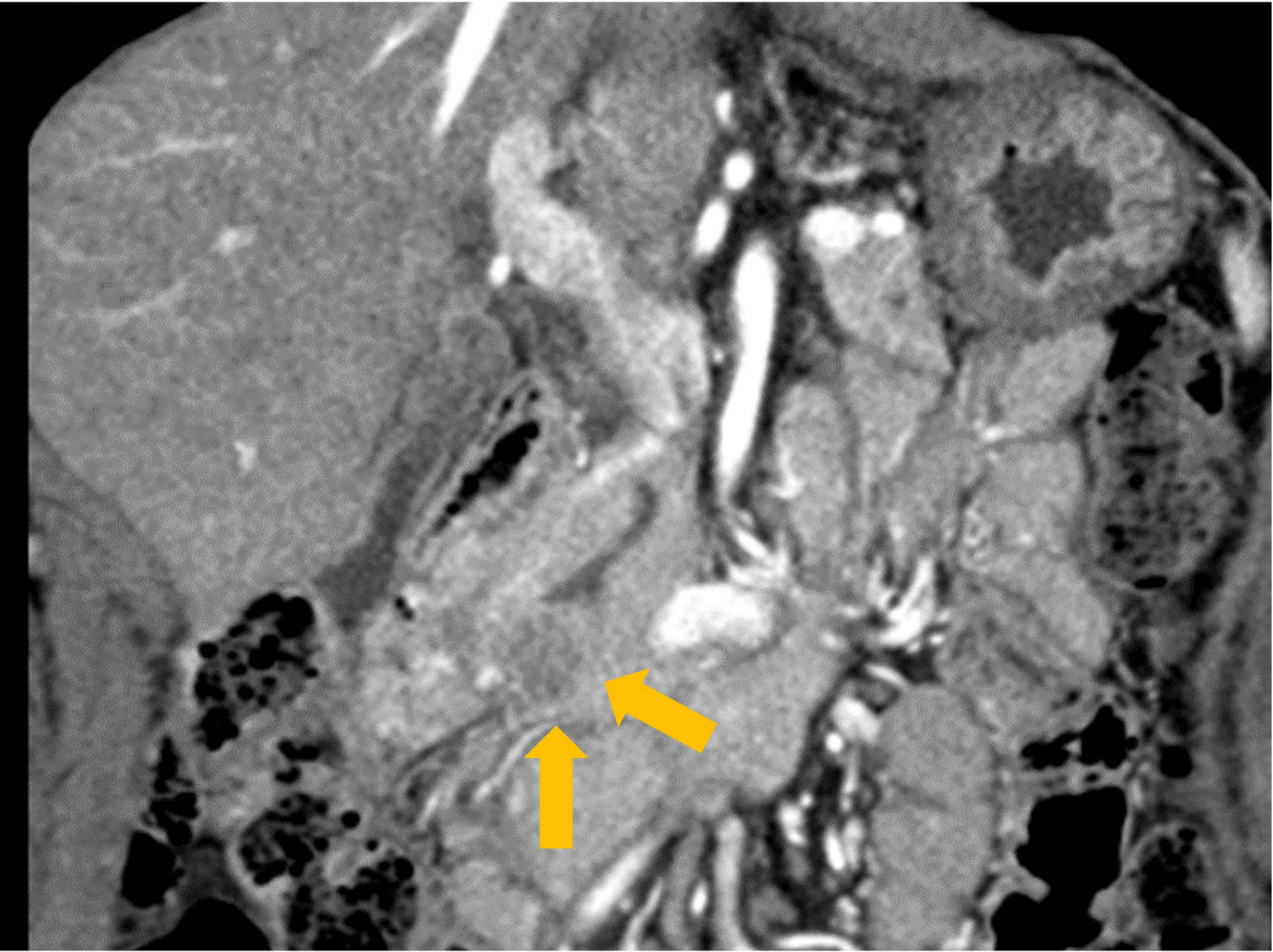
Contrast CT (coronal) There is a 20 mm hypovascular tumor in the pancreatic hook area along with stenosis of the main pancreatic duct at the same site.
